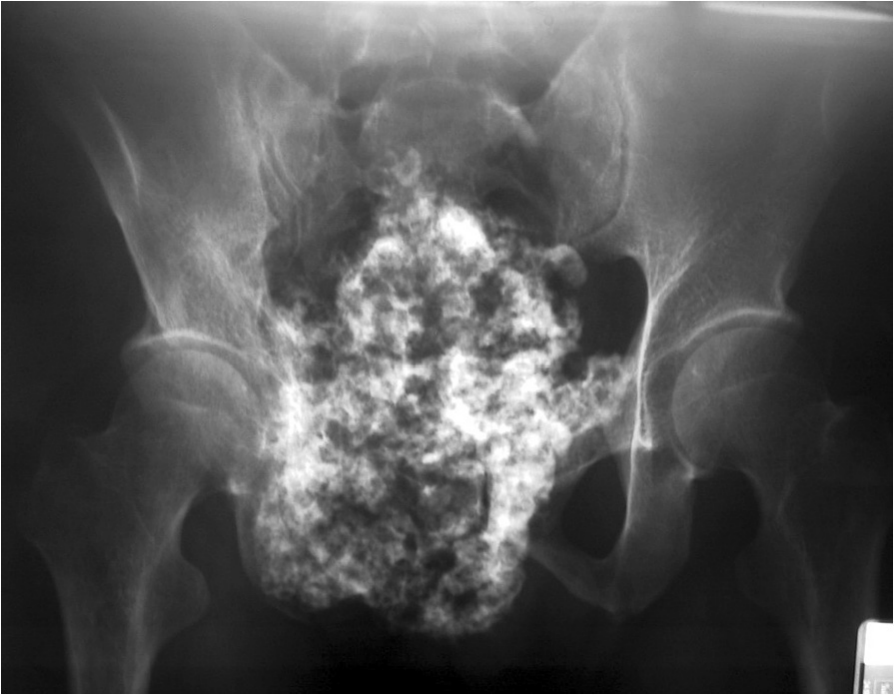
Anteroposterior radiograph of the pelvis showing chondrosarcoma is located at the right pubis and periacetabulum and extends to the left pubic area.
